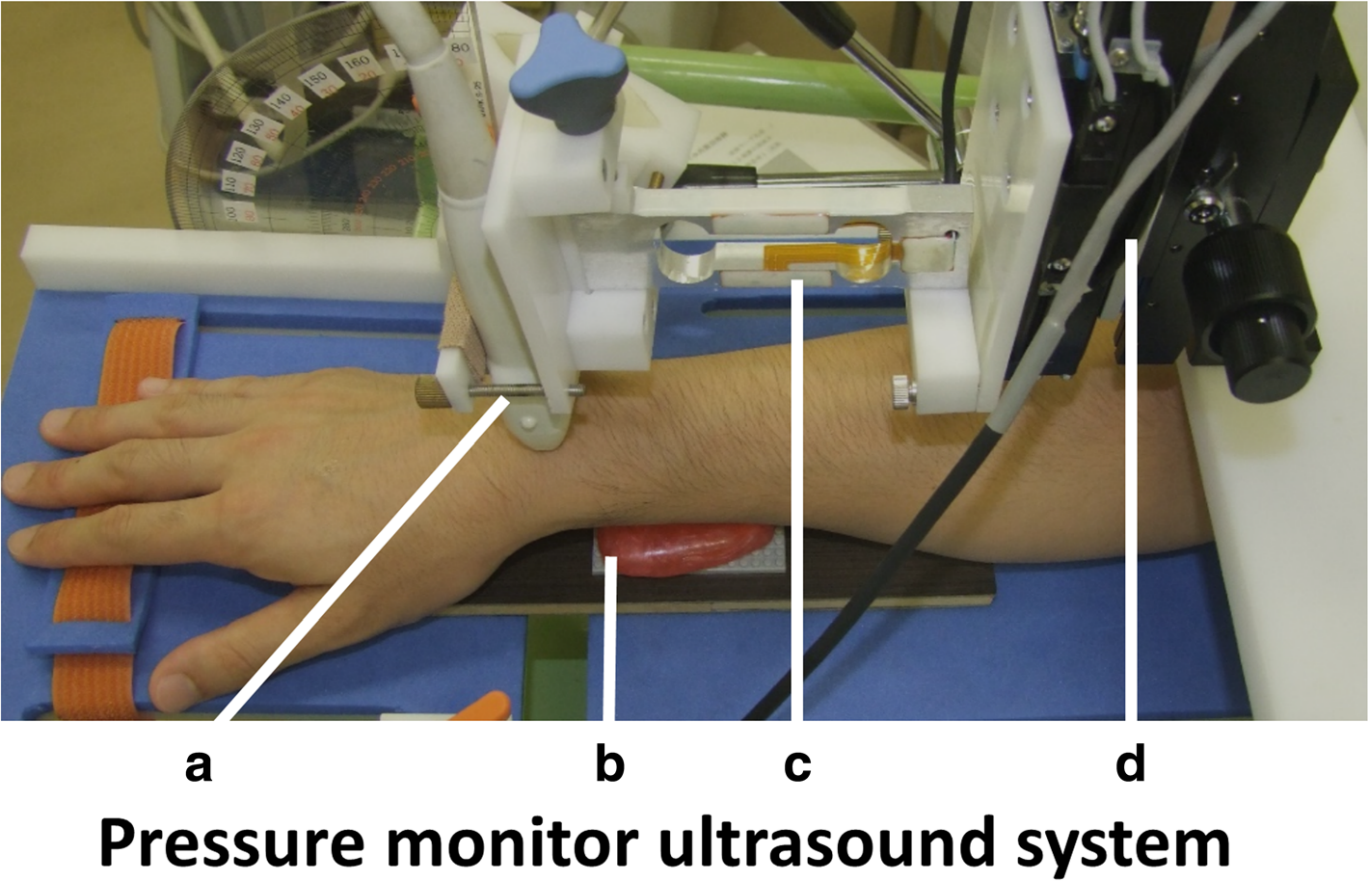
Pressure-monitor ultrasound system and the setup for the measurements. The pictures show the forearm position. The pressure-monitor ultrasound system was developed to apply pressure to the tissue with a pre-determined cycle and transducer displacement. a Transducer, b block, c pressure sensor, and d motor
